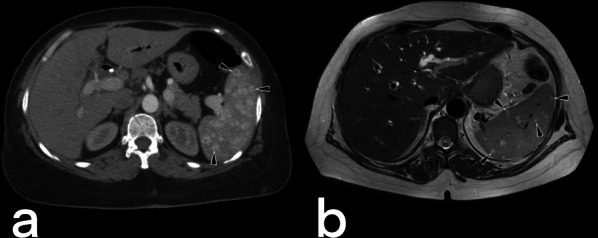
Axial contrast-enhanced CT (a) and axial T2 weighted MR (b) images of a 66-year-old female demonstrate multiple foci (arrowheads) in the spleen. Note small hypodense areas with peripheral enhancement in foci (a). Splenic TB
